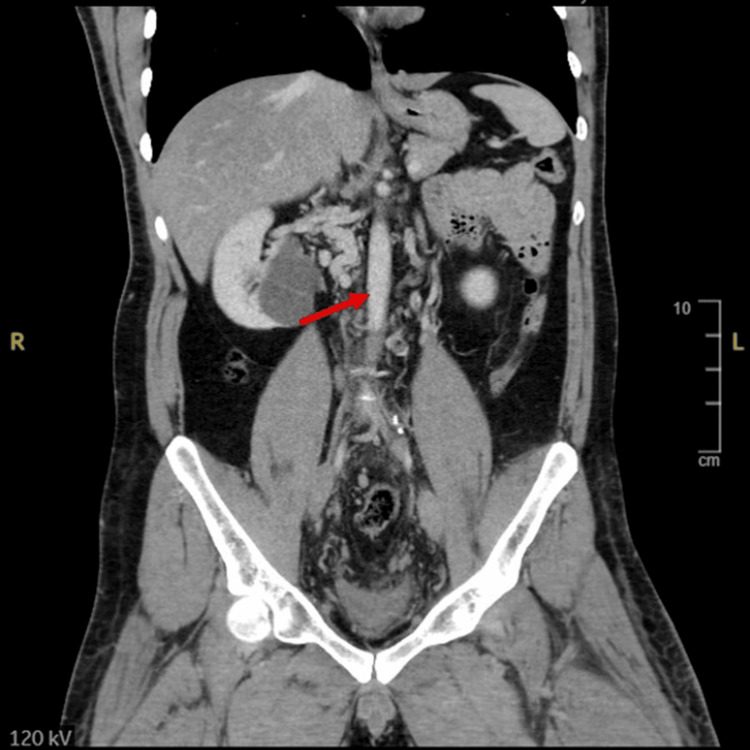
Contrast-enhanced CT of the abdomen demonstrating absence of the inferior vena cava with extensive collateralization CT: computed tomography
